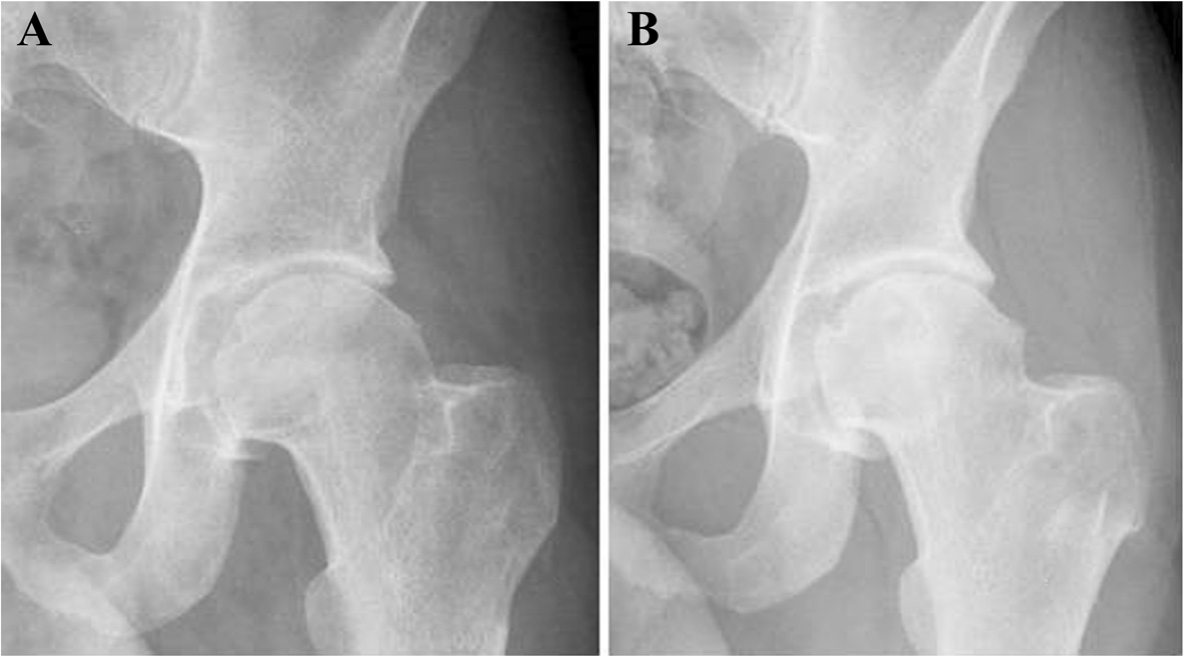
Initial and follow-up radiographs of the left hip of a 31 year-old male with acetabular osteoid osteoma. a Plain x-ray of the left hip before undergoing radiofrequency ablation or hip arthroscopy showing mild degenerative changes of the hip joint; b Plain x-ray of the left hip of the same patient 44 months following hip arthroscopy and radiofrequency ablation of the acetabular osteoid osteoma not showing further progression of the osteoarthritic changes
